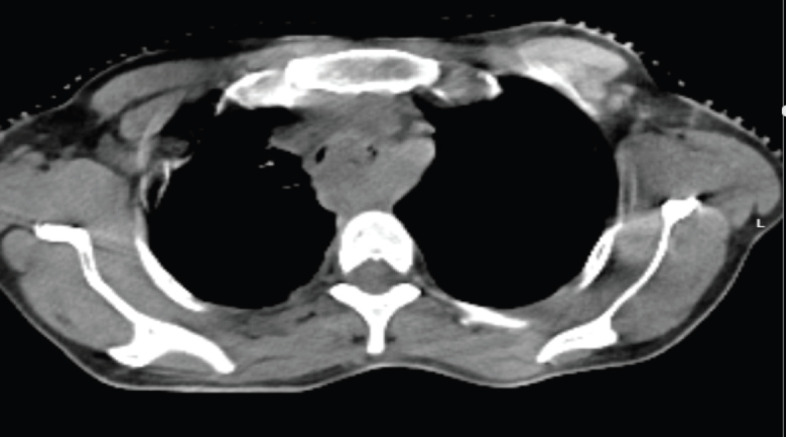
Axial CT when she presented with symptoms of dyspnea, showing MCAO.
